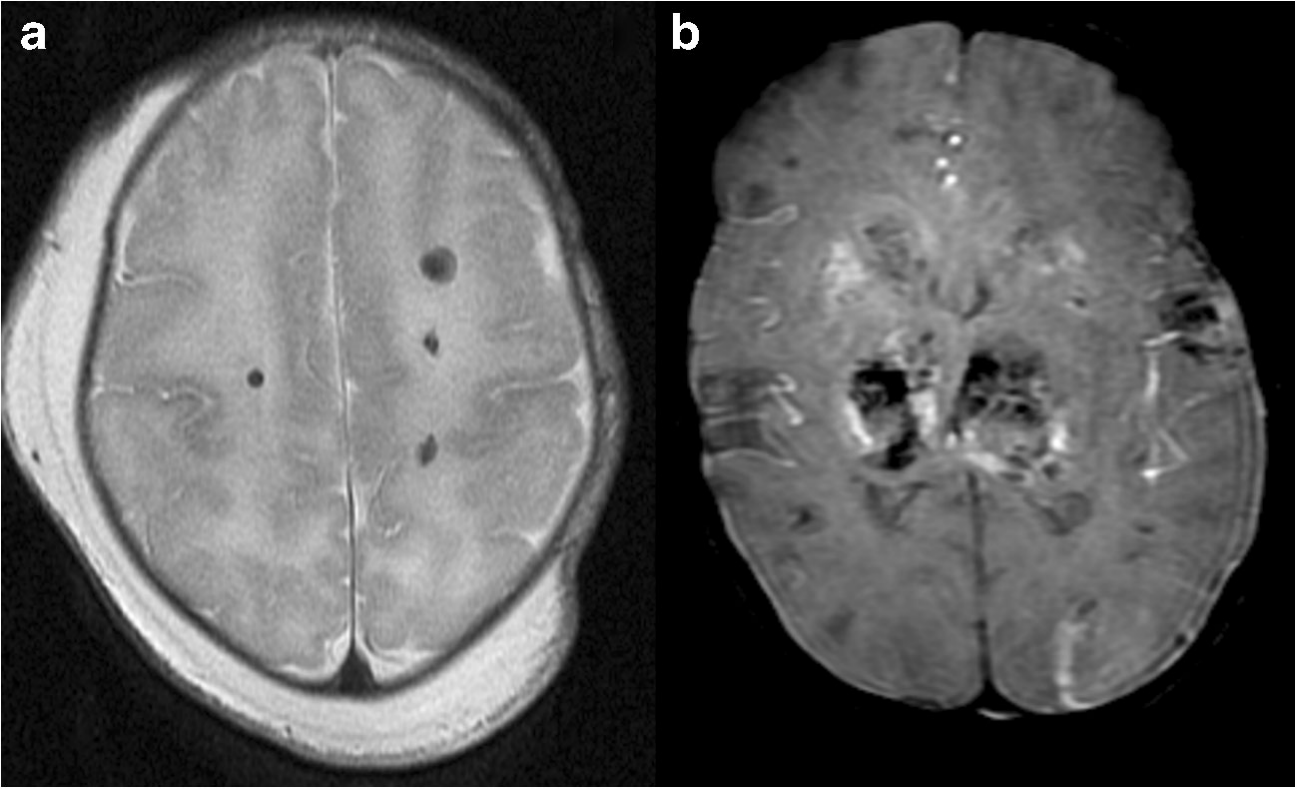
Axial T2-weighted MR image (a) at the level of the deep white matter and axial SWI MR image (b) at the level of the thalami. Focal hemorrhagic lesions are shown in the deep white matter (a) and more confluent hemorrhagic foci are demonstrated in both thalami (b)
